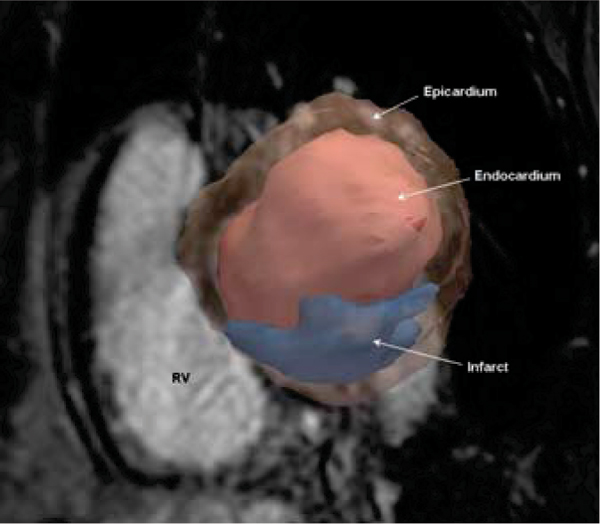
3D rendering of LV and infarct (infarct mass: 14.1 g, iSA: 52.9 cm^2^, iSA/V: 3.9 cm^2^/ml) superimposed on a basal LV short-axis DE-MR image.
